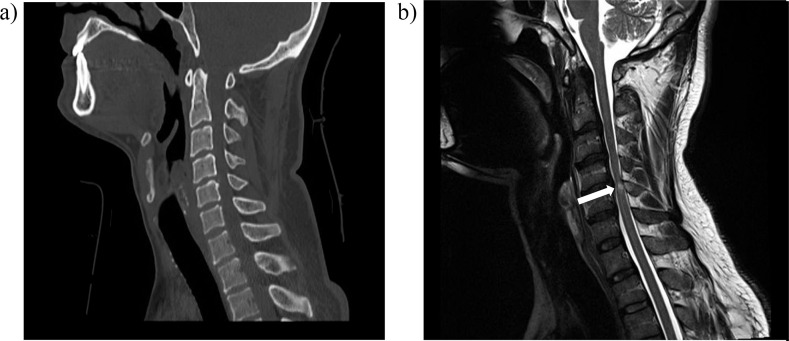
A case of SCIWORA. (a) Normal computed tomography of the cervical spine. (b) tbl1- and tbl2-weighted magnetic resonance imaging of the cervical spine (same patient) with spinal cord contusion (white arrow)
